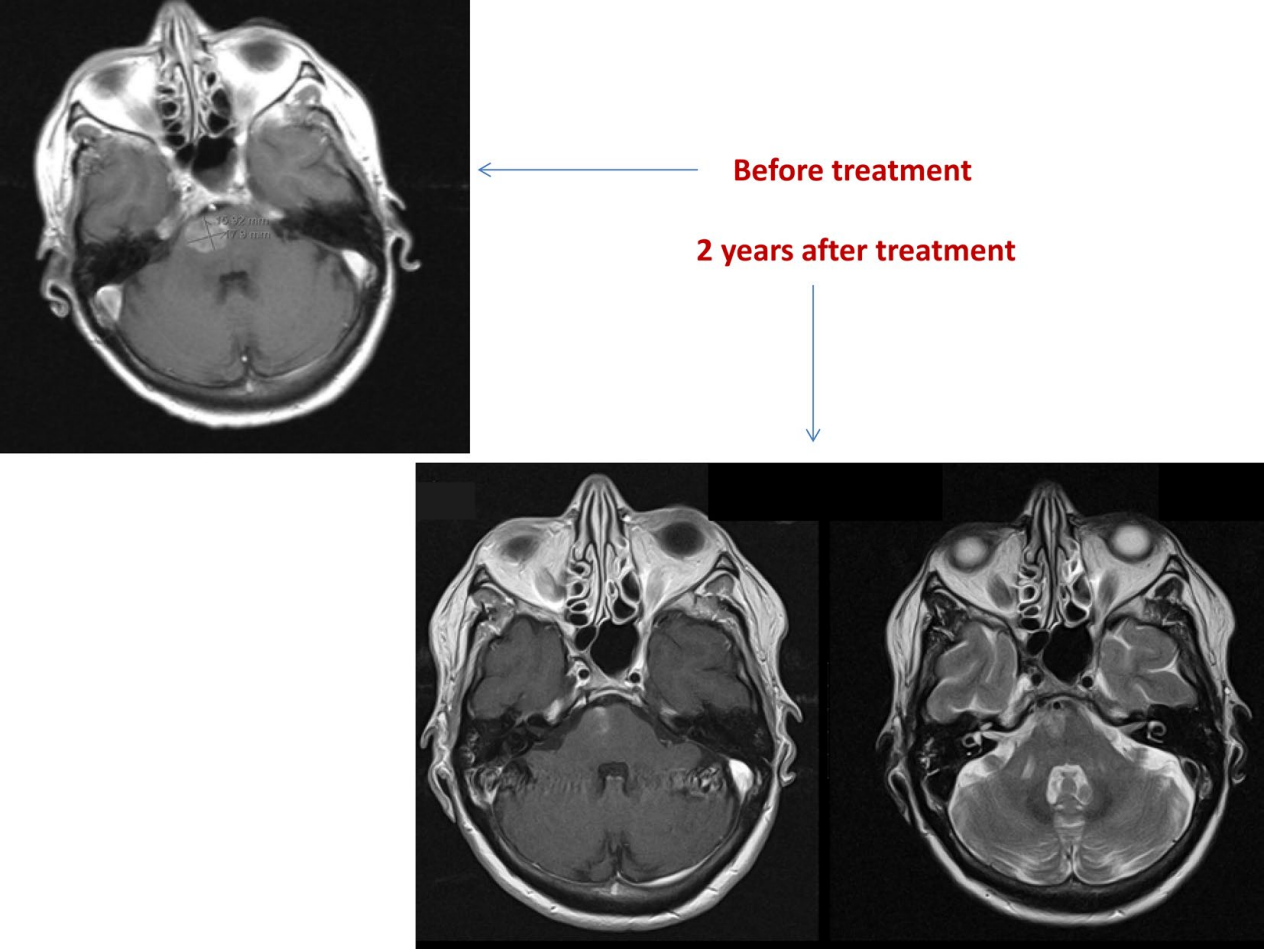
Magnetic resonance images before stereotactic fractionated radiotherapy in a 61-year old patient with solitary metastasis in the brain stem, HER2 positive disease, excellent performance status. The lesion received 7 fractions of 5 Gy prescribed to the 80 % isodose line. Two years later, radiation-induced changes developed, which resolved after treatment with steroids and pentoxifylline. The patient is alive without relapse
